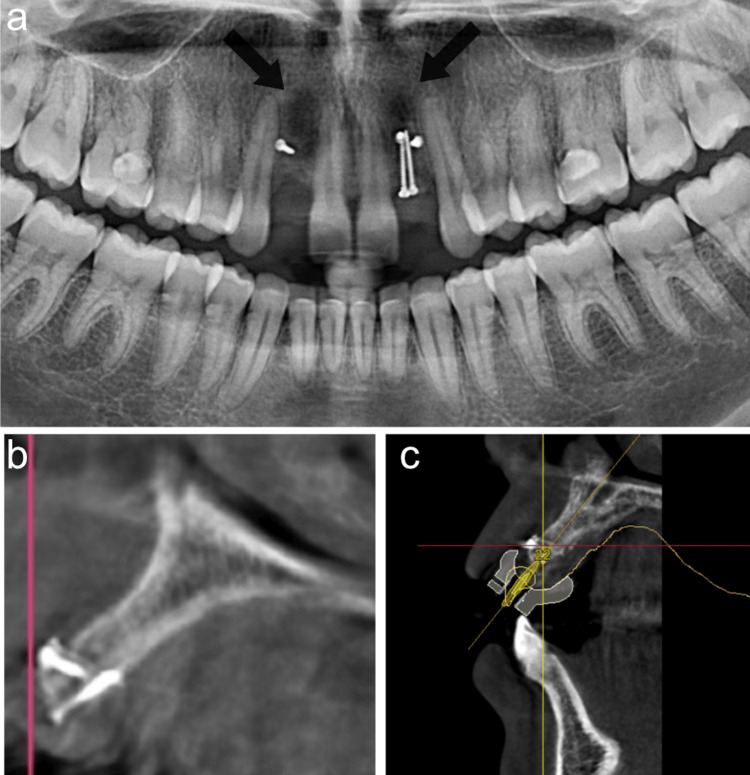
Postoperative radiographic image documenting the reconstructed alveolar bone after surgical healing: a) panoramic radiograph; b) and c) cross-sectional views.
